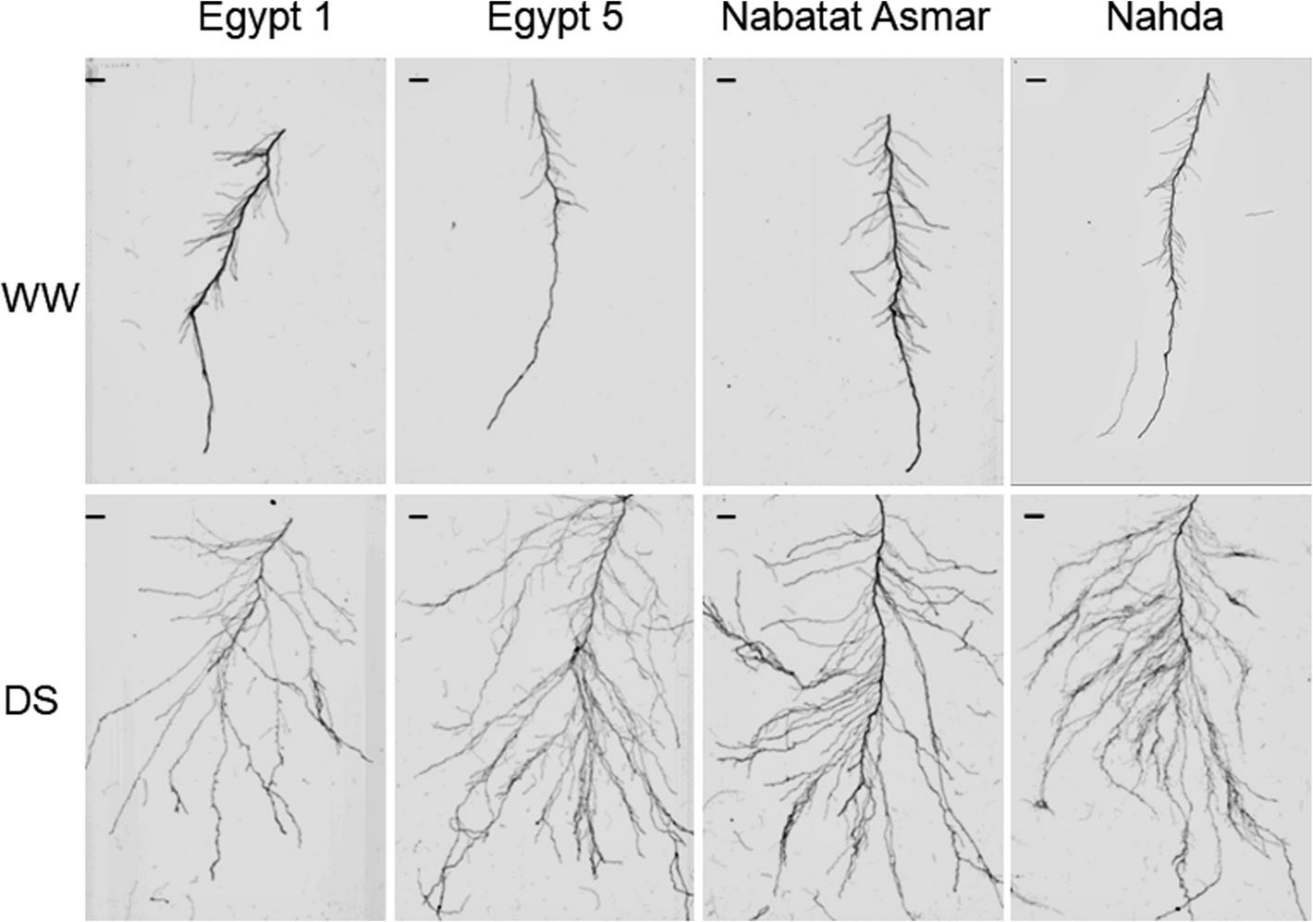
Representative images of the apical 20 cm of nodal roots from well-watered (WW) and drought stressed (DS) plants. Scale bar represents 10 mm
